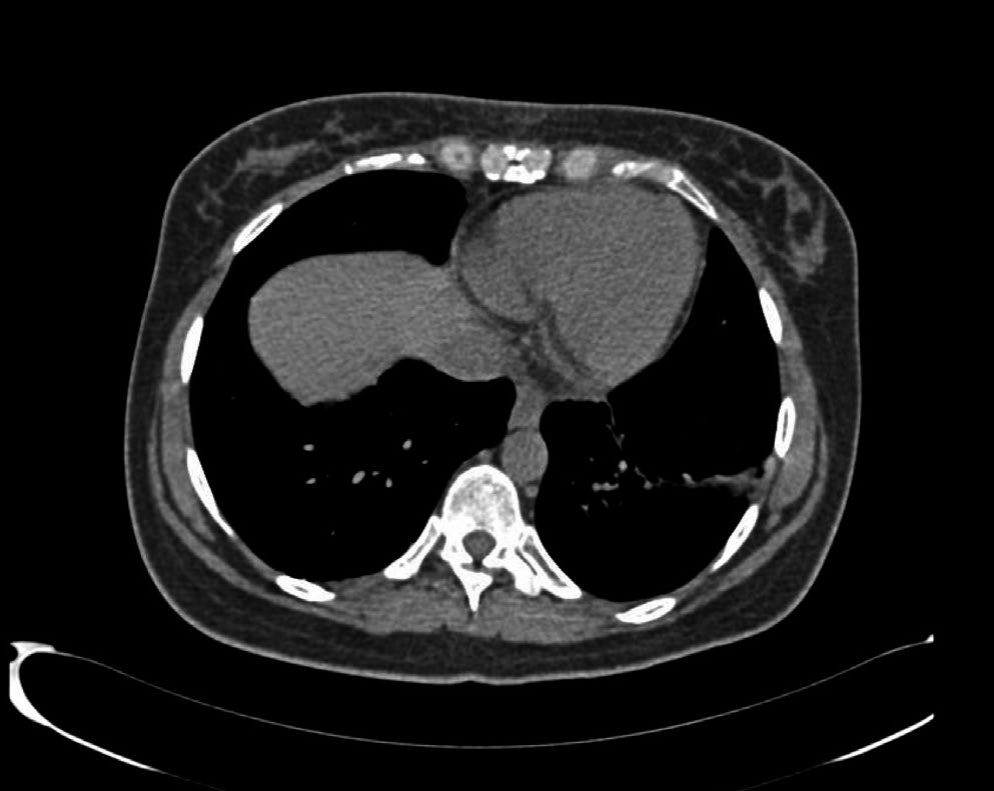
Follow‐up CT scan showed resolution of the left‐sided pleural effusion
